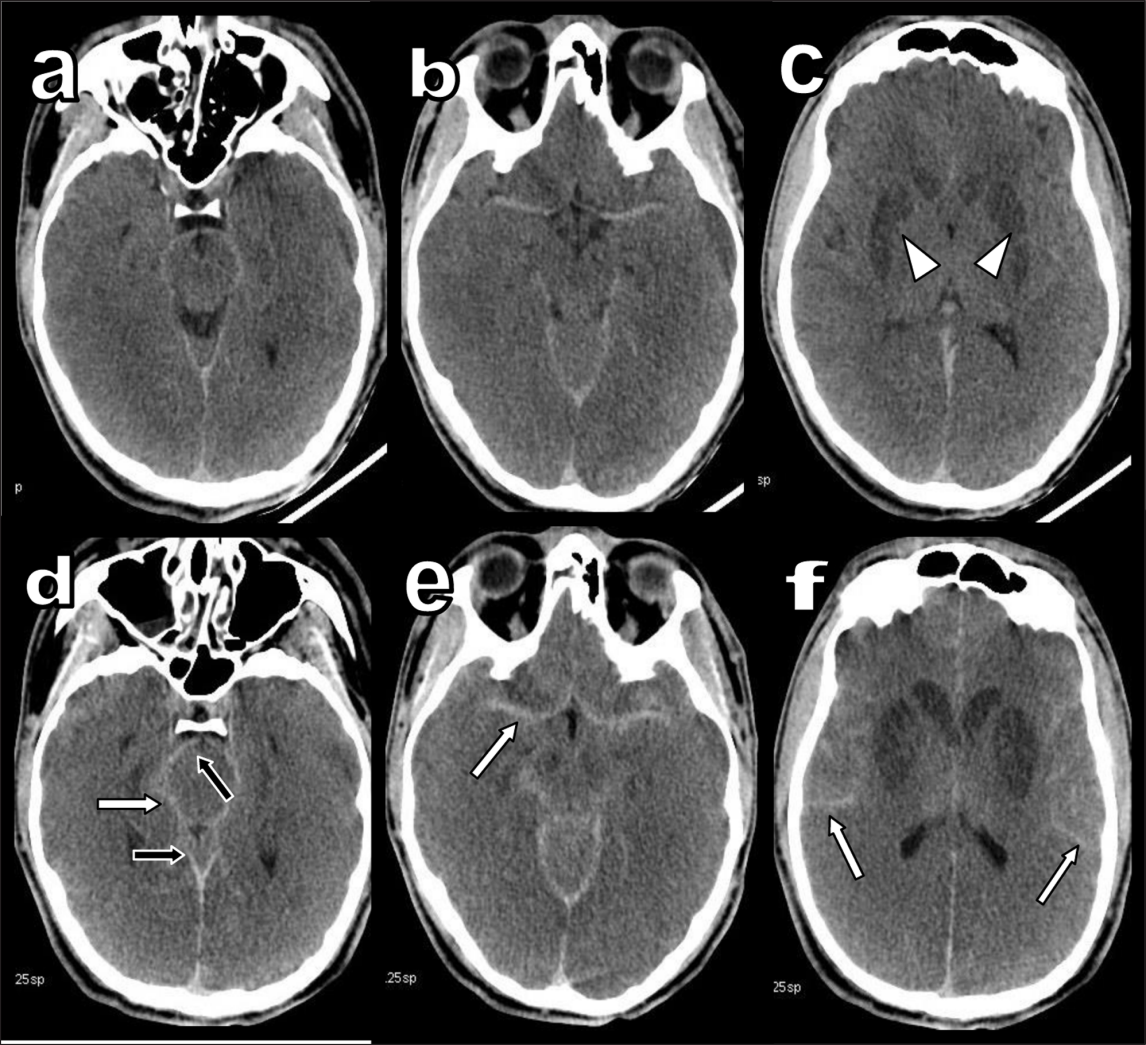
Comparison of unenhanced Brain CT (UBCT) obtained 5 hours after admission (a to c) and 30 hours after admission (d to f).
